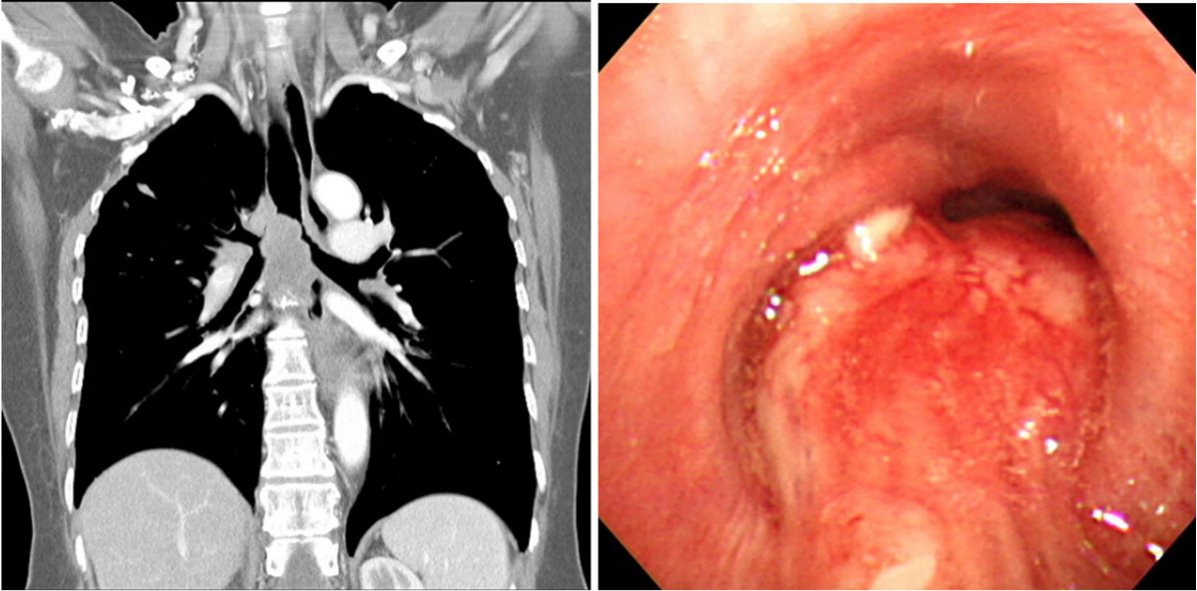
The chest CT and fiberoptic bronchoscopy show mass in carina and right main bronchus.
